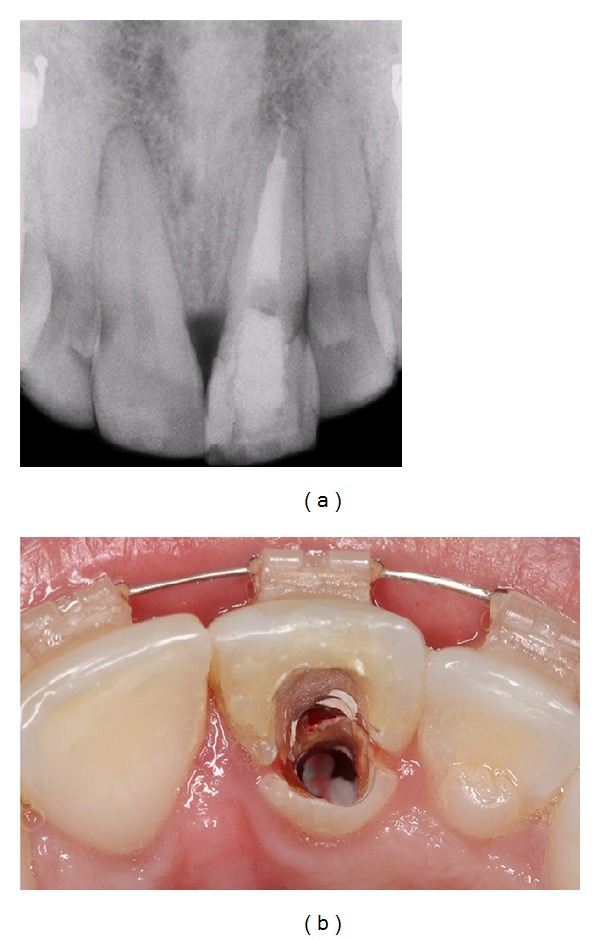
Radiographic and clinical images showing the endodontic retreatment, with the installation of an intracanal pin and temporary crown placement.
